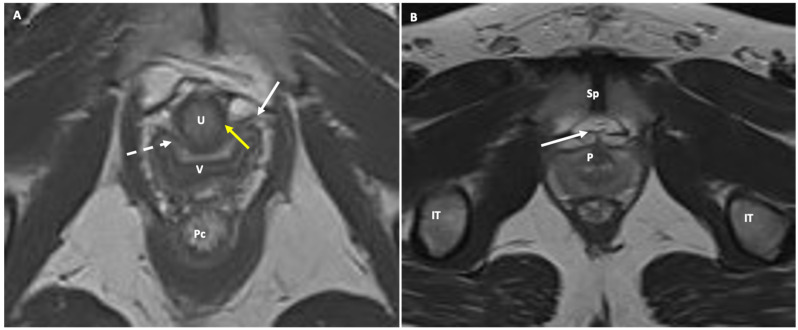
Axial T2-weighted MR images of levator ani hiatus showing the periurethral (white arrow), the paraurthral (yellow arrow), and pubourethral (white dotted line) female ligaments (A). Corresponding similar structures can occasionally be seen in young males (B) in the retropubic space just ventral to the prostate (arrow). U: urethra; V: vagina; Pc: pubococcygeal muscle; Sp: symphysis pubis; IT: ischial tuberosity; P: prostate.
